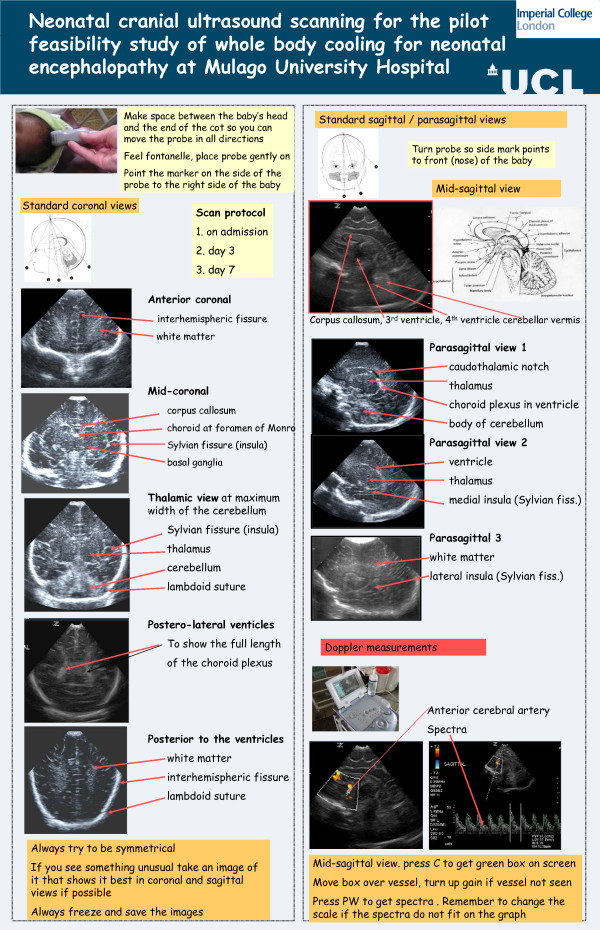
Neonatal cranial ultrasound scanning for the pilot feasibility and safety study of a simple, low cost method of whole body cooling for neonatal encephalopathy at Mulago Hospital, Kampala
